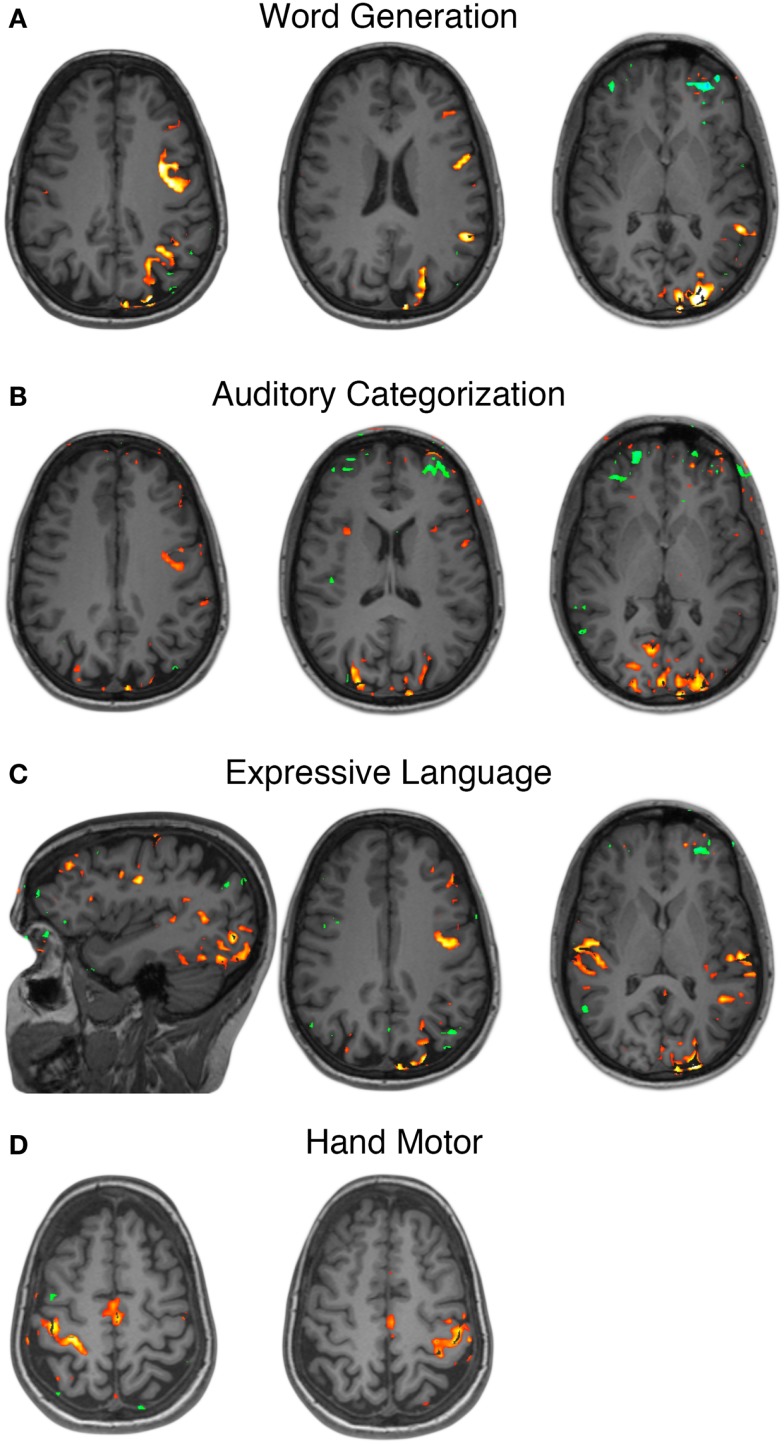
Functional MRI localization of speech and motor cortex. Functional MRI for various language tasks revealed left sided dominance as well as recruitment of posterior occipital lobe cortical areas (A–C). Unilateral hand motor tasks revealed primary motor cortex activations in the contralateral pre-central gyrus (D).
